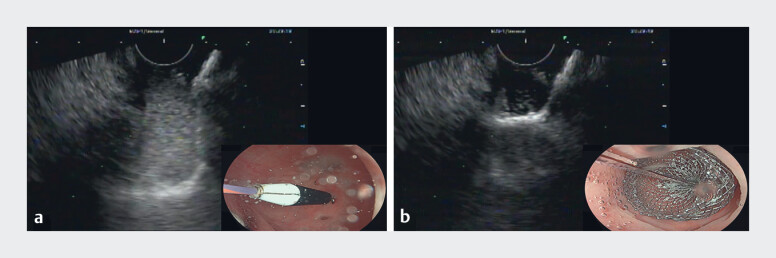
Endosonographic and endoscopic images of LAMS deployment.
